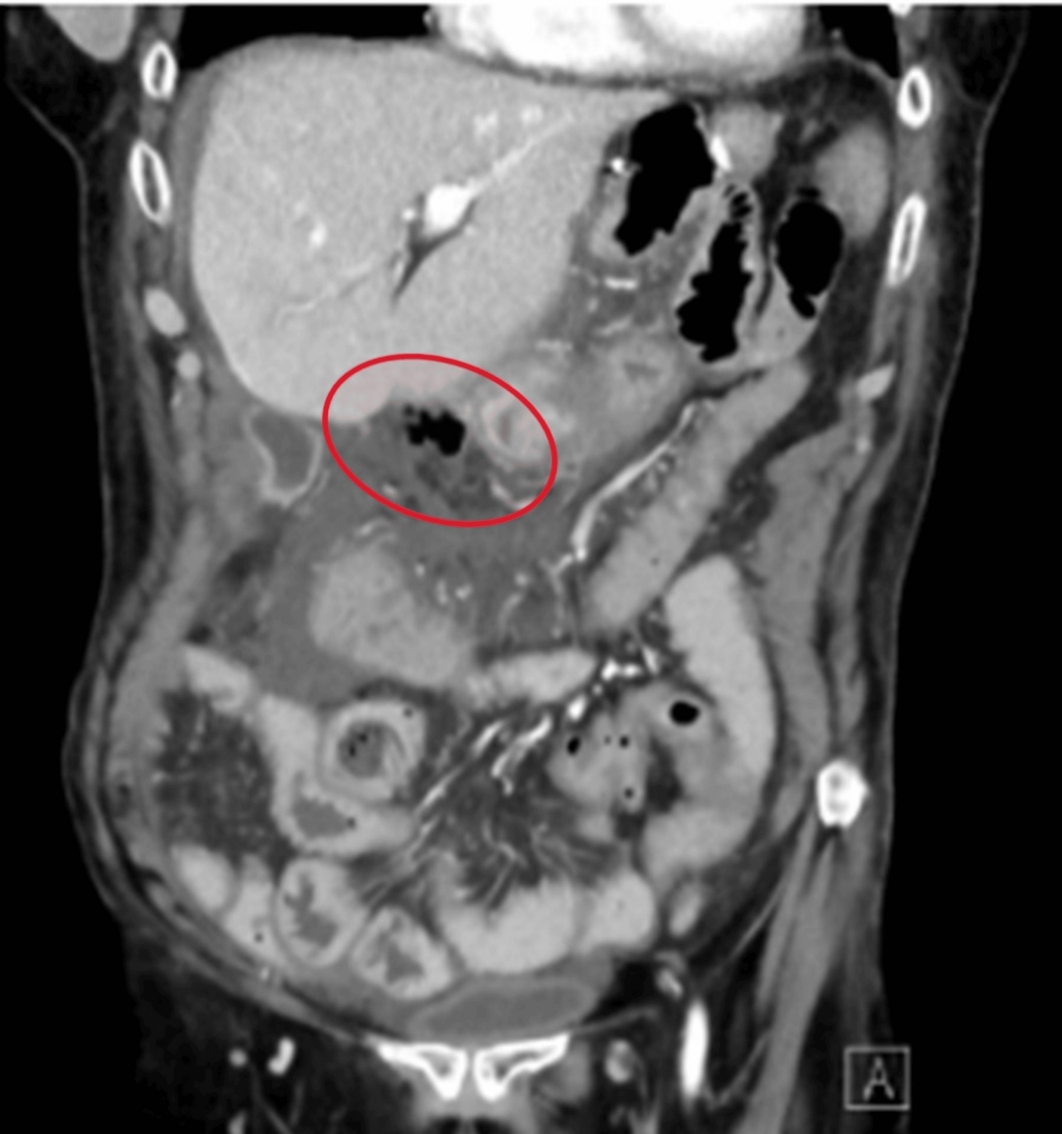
A CT of the abdomen and pelvis with the presence of free air in the abdomen near the duodenum
